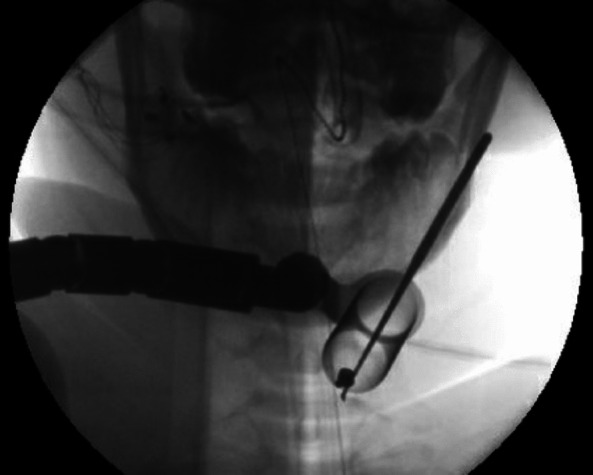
Intraoperative AP X-ray showing pellet lying within working channel
